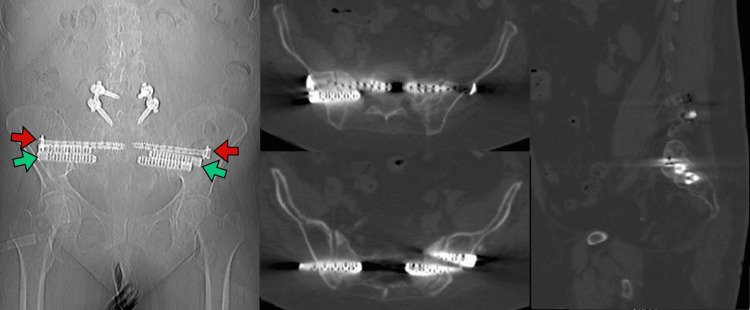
Details the surgical treatment received, including a panoramic X-ray (first image) that shows bilateral trans-sacral iliac screws (red arrows) and two screws for bilateral sacroiliac fusion (green arrows). Both axial and sagittal CT images (second and third images) confirm correct screw placement.
